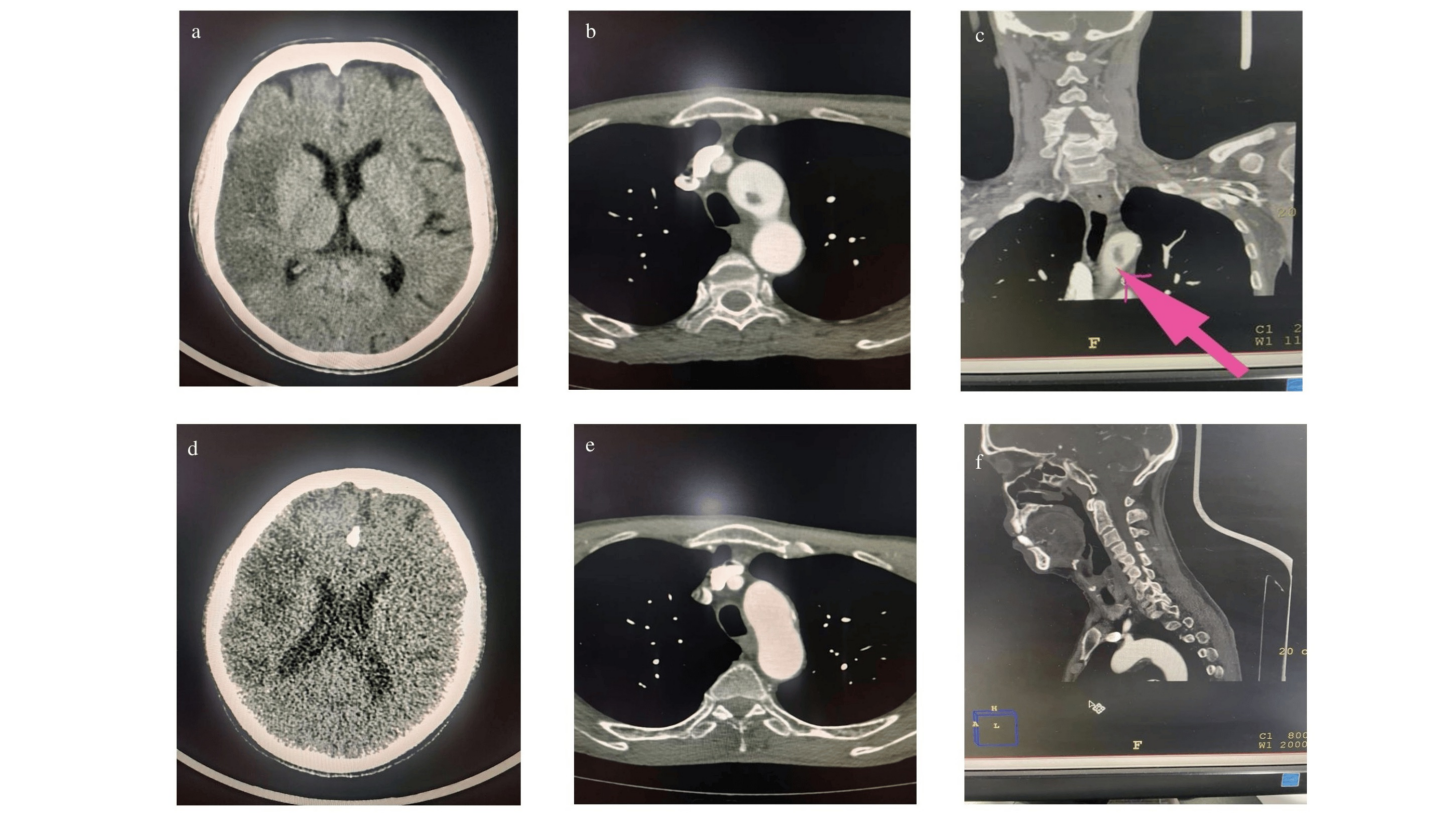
a. NCCT of the head suggestive of right fronto-temporoparietal acute infarct; b and c. Axial and coronal CT angiography sections showing a hypodense filling defect arising from the inferior wall of the aortic arch, consistent with free-floating thrombus (pink arrow); d. NCCT of the head performed on follow-up showing a near-stable infarct; e and f. Axial and coronal CT angiography sections showing no filling defect, suggestive of thrombus resolution. NCCT, non-contrast computed tomography; CT, computed tomography
